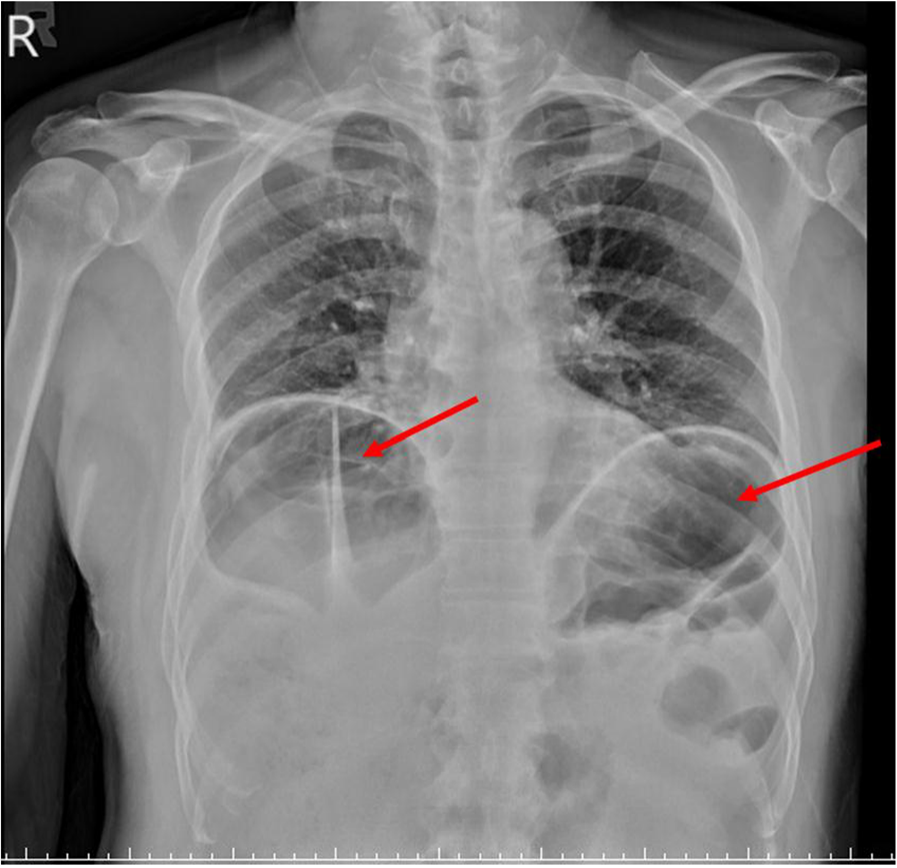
Plain film of chest. Chest radiography showed right fifth to eighth rib fractures and was suspicious for free air under our patient’s bilateral hemi-diaphragm (red arrows).
